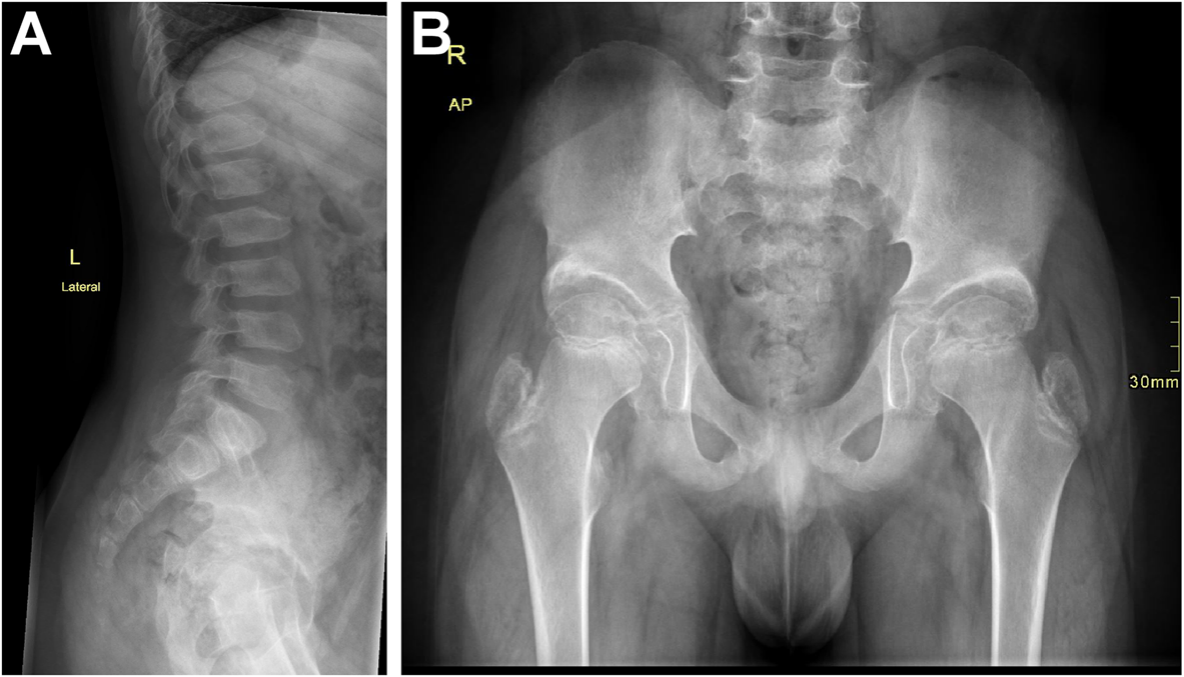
a: Spine radiograph showing generalized decreased bone density and flat vertebral bodies with multiple anterior beaking. b: There was involvement of both hip joints, with enlarged femoral epiphyses and narrowing of the hip joint space. Medial femoral flattening of the femoral heads with a short femoral neck causing bilateral coca valga was also noted. Marked arthritic changes were present affecting both sides of each joint, with subcortical erosion, subcortical cysts, and narrowing of the joint spaces
